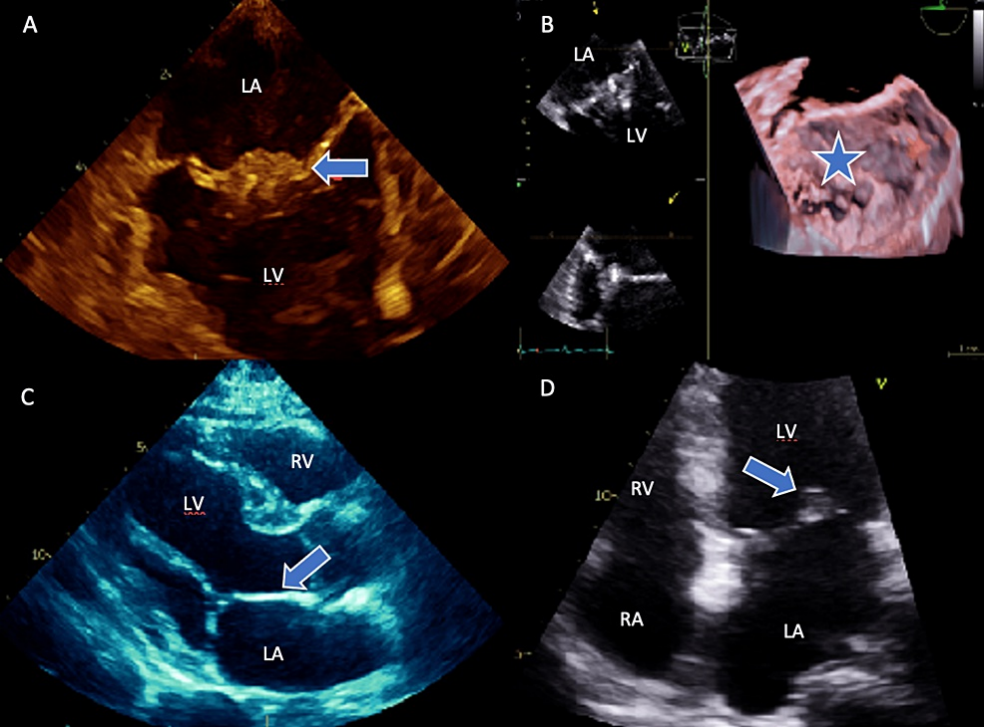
Transesophageal echocardiography with thrombus identified (panels A & B) in comparison to absent findings on transthoracic echocardiography (panels C & D) Panel A- TEE in commissural view showing a large 1.0 x 0.8 cm heavily dense mass consistent with thrombus located on the atrial side of the anterior mitral valve leaflet (arrow). Panel B- TEE 3-D image showing a large echogenic density located on the anterior leaflet of the mitral valve (star). Panel C- TTE in parasternal long axis view which shows no mitral valve pathology (arrow showing anterior mitral valve leaflet with no thrombus). Panel D- TTE in apical four chamber view which shows mildly thickened anterior leaflet of the mitral valve (arrow), otherwise no vegetation or thrombus. TTE: Transthoracic echocardiography, TEE: Transesophageal echocardiography, LA: left atrium, LV: left ventricle, RA: right atrium, RV: right ventricle
